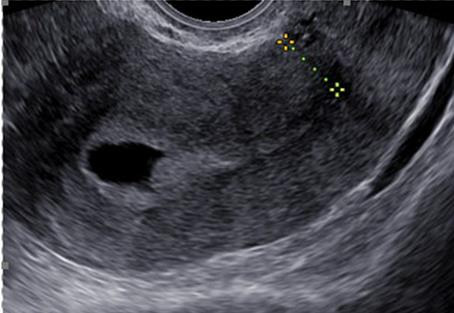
Postoperative ultrasound imaging.
